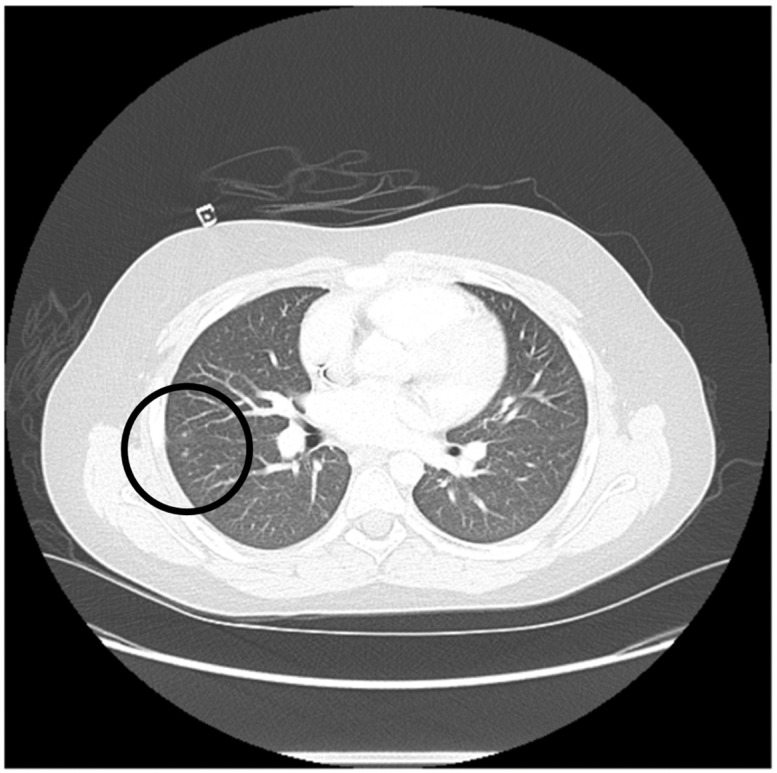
Computed tomography (CT) chest imaging of an 11-year-old patient with relapsed AML is shown. CT chest imaging with bilateral ground-glass opacities and pulmonary nodules (seen inside the black circle) consistent with pulmonary toxoplasmosis. The CT chest was performed 3 months prior to HSCT.
